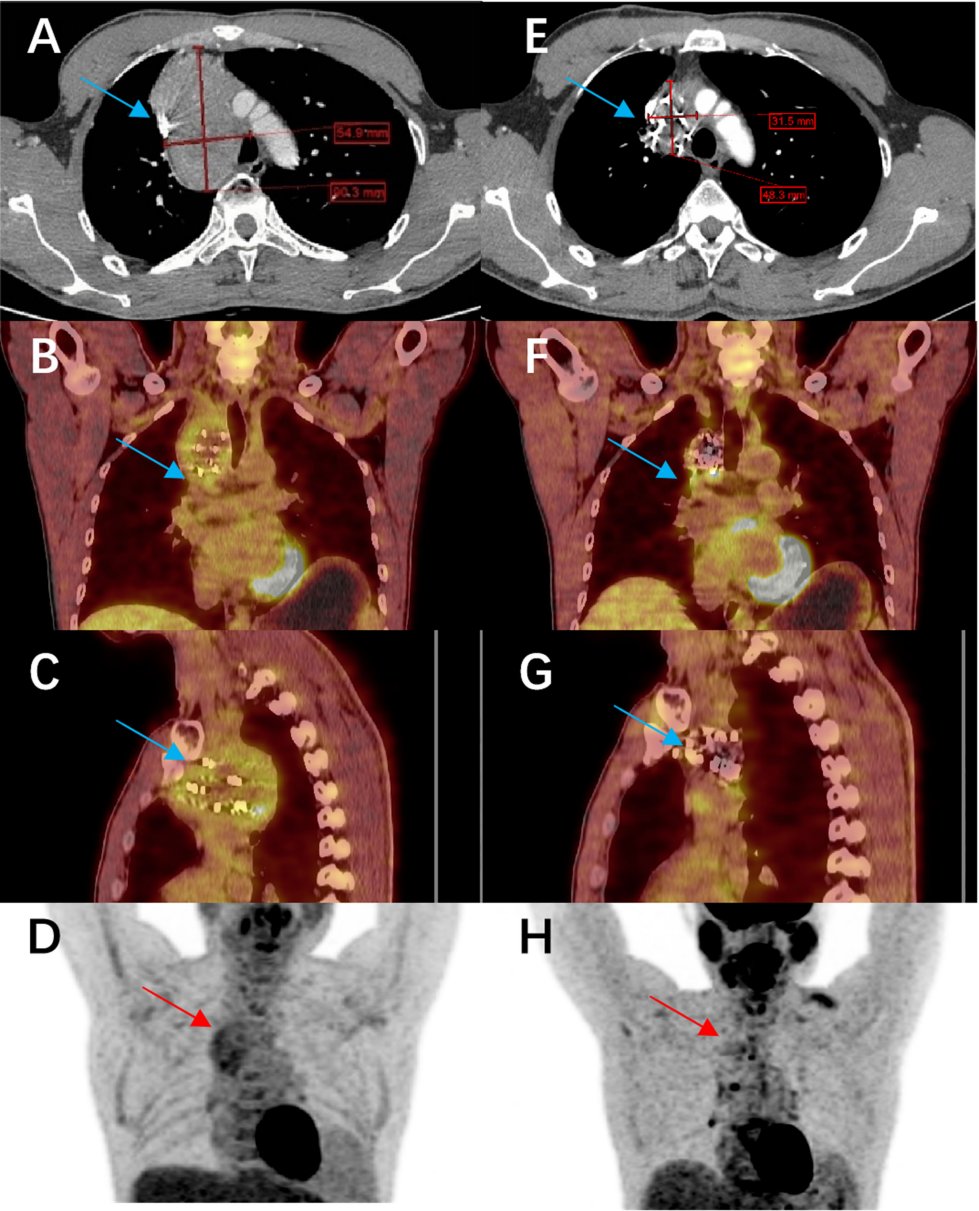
Comparison of imaging before and after treatment with anlotinib combined with ^125^I seed implantation in a patient with refractory papillary thyroid carcinoma. The patient had been on anlotinib since March 2021, and a total of 140 seeds were implanted. (A–D) show the pre-treatment CT and PET-CT images of the lesion (cross-sectional views), and (E–H) show the corresponding post-treatment CT and PET-CT images. The lesion has significantly shrunk, and its metabolic activity has markedly decreased.
